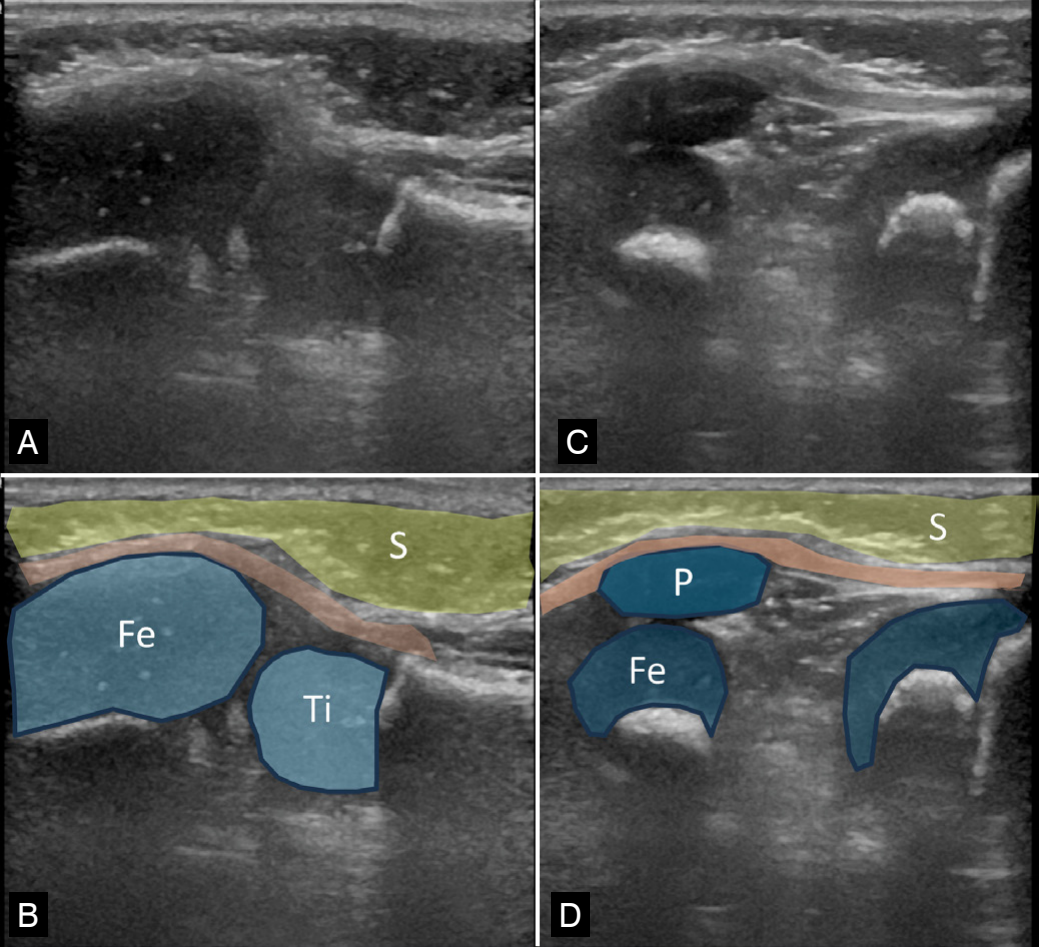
Male patient, four months old, diagnosed with right fibular hemimelia and knee dislocation. The patella was not palpable, and ultrasound confirmed the diagnosis of agenesis of the right patella (A). On the left side (C), the patella is present, and the longitudinal section shows the patella. In B and D, the subcutaneous tissue is marked in yellow, the epiphyseal cartilages in blue, and the extensor mechanism in orange; Fe = femur, P = patella, S = subcutaneous tissue, Ti = tibia.
